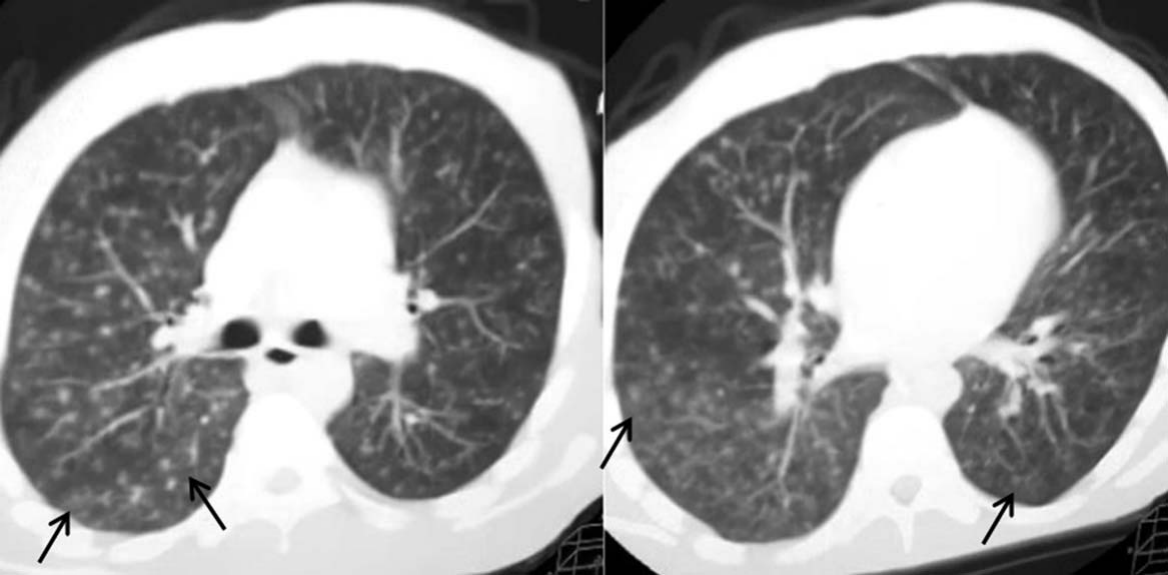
Chest computed tomography; diffuse miliary and ground-glass opacities (arrows).
